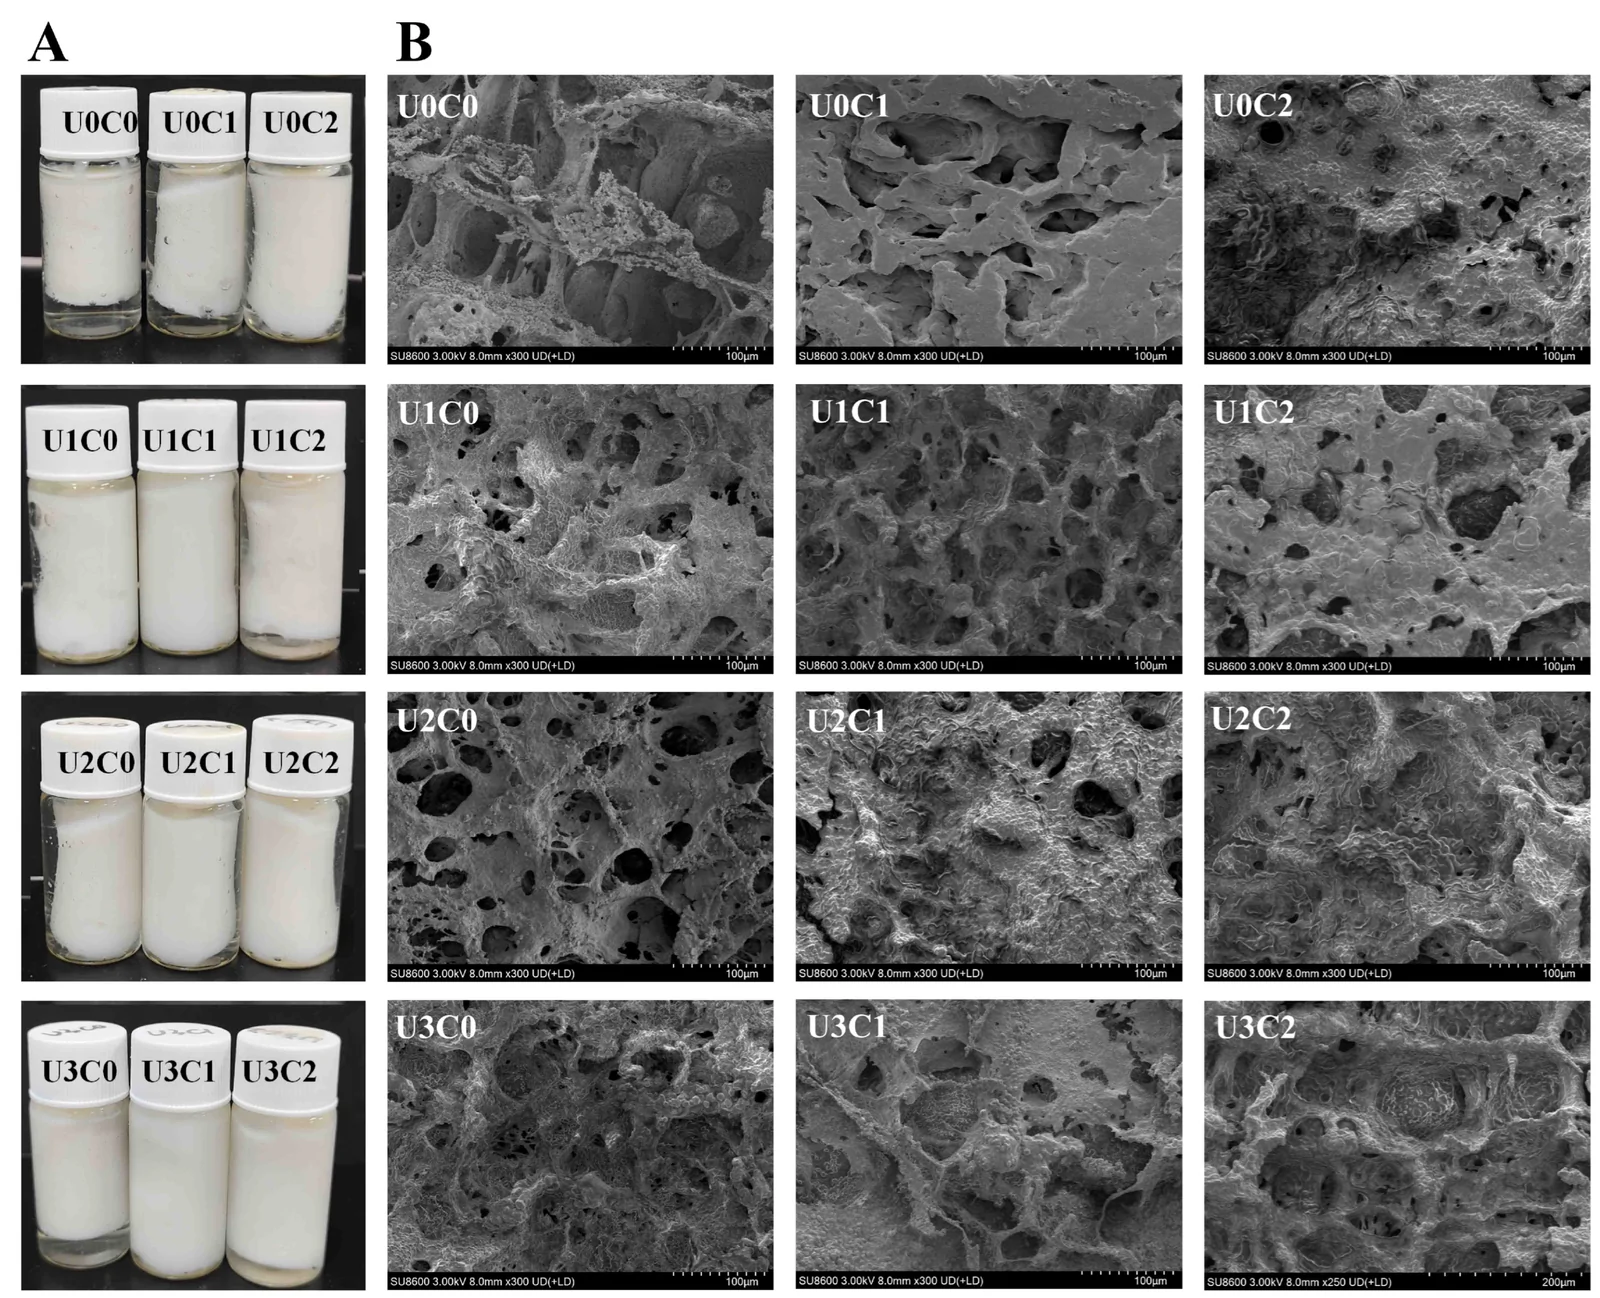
Effects of different concentrations of CSO combined with different ultrasonic power levels on the (A) macroscopic morphology and (B) microstructure (SEM images) of yak meat MP gels.
